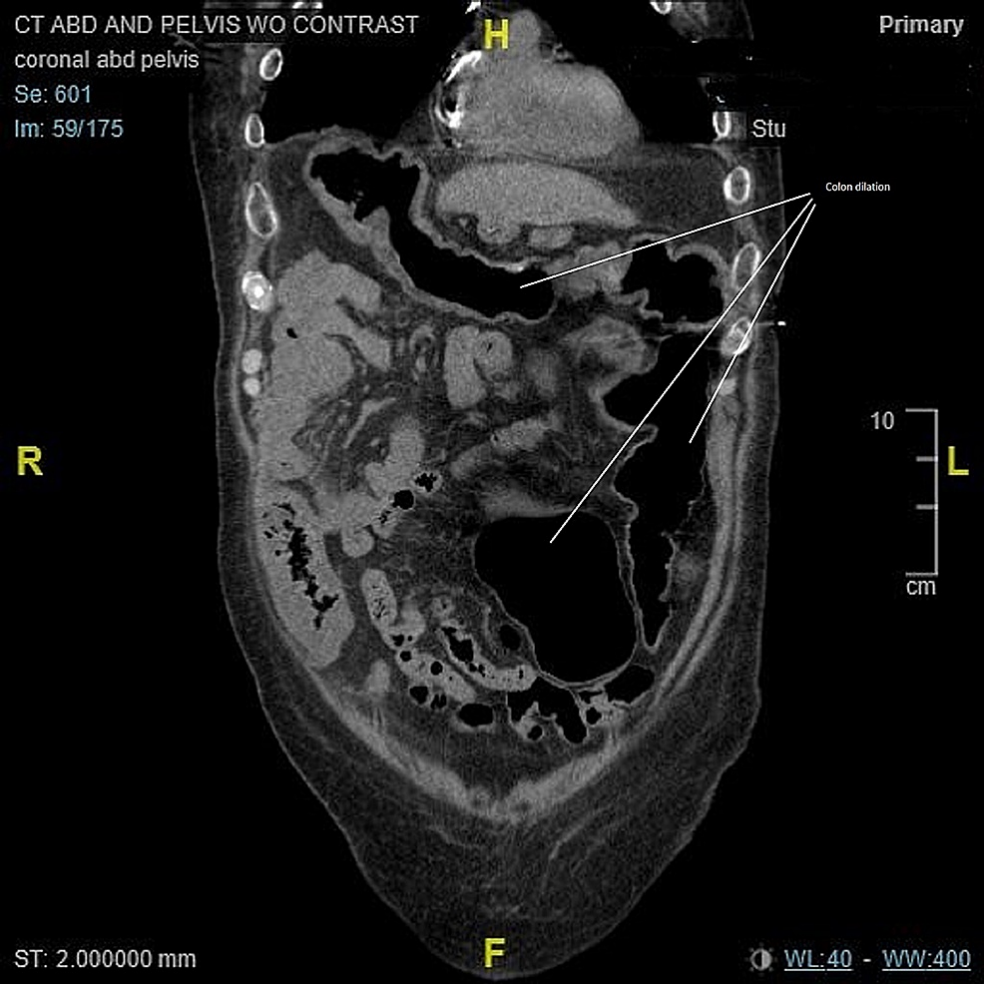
CT of the abdomen/pelvis on worsening of infection. Coronal view: Circumferential thickening of the colon wall most marked within the right colon and increased in the transverse colon. Increased colonic wall thickening concerning for progression of infection. Before the use of fidaxomicin. CT: computed tomography
